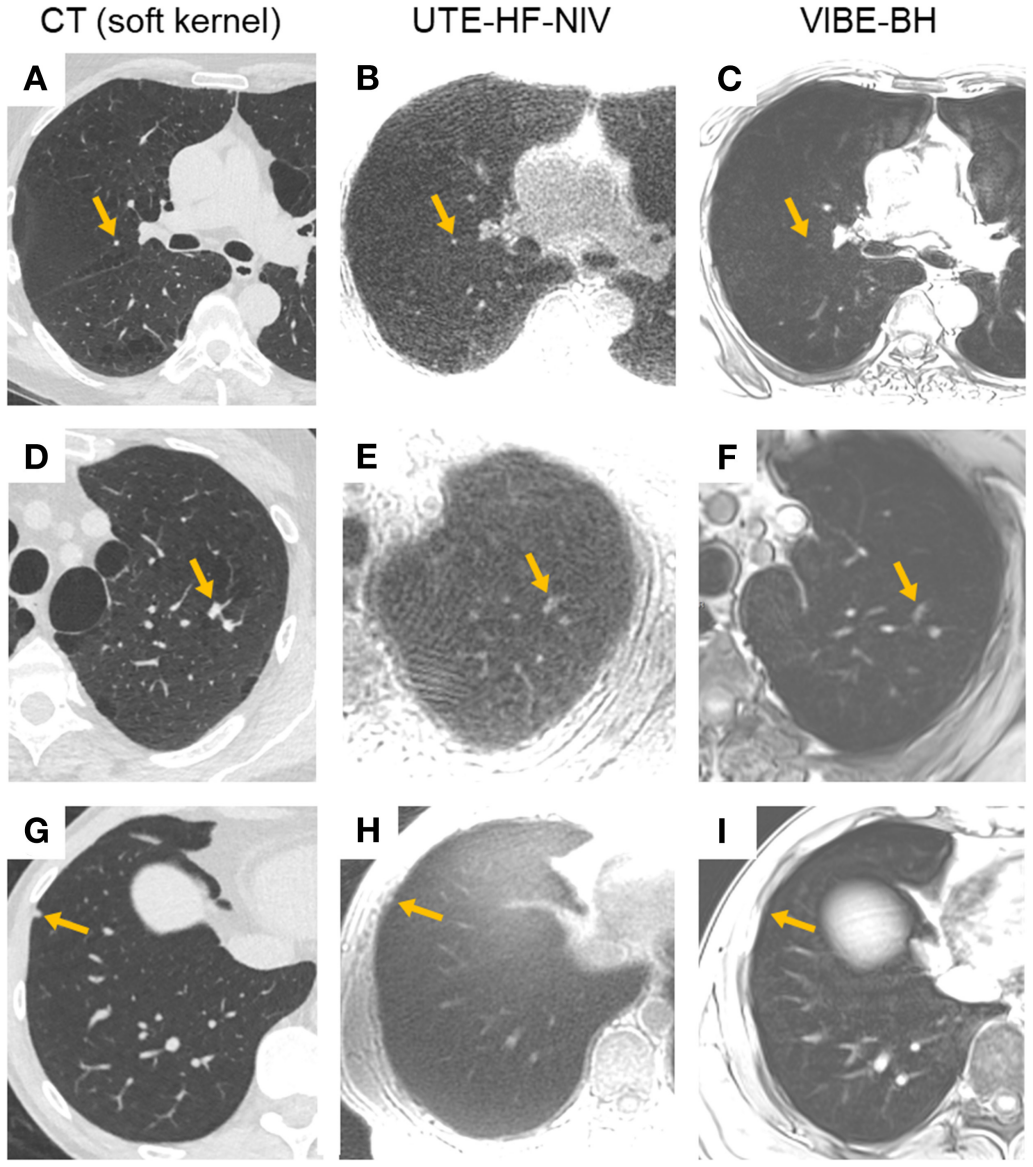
Examples of nodules not reported in MR analyses. (A–C) CT, UTE-HF-NIV, and VIBE-BH images of the right upper lobe showing a juxta-fissural nodule of 4-mm long axis (orange arrow), respectively. While visible in UTE-HF-NIV image (B), its long axis was measured <4 mm, hence not reported. The nodule was scarcely seen in (C). (D–F) CT, UTE-HF-NIV, and VIBE-BH images of the left upper lobe with 2 nodules of 9 mm (orange arrow) and 6-mm-long axis, respectively. Due to a loss in morphological characteristics in both MR images, and although the neighboring nodule was easily recognized, the largest finding was considered as a parenchymal band instead of a nodule for two of the three readers, and as a nodule for the third reader. (G–I). CT, UTE-HF-NIV, and VIBE-BH images of the right lower lobe with a nodule of 5-mm long axis (orange arrow), respectively. The nodule was barely seen on UTE-HF-NIV image (H) and reported as absent in both MR images.
